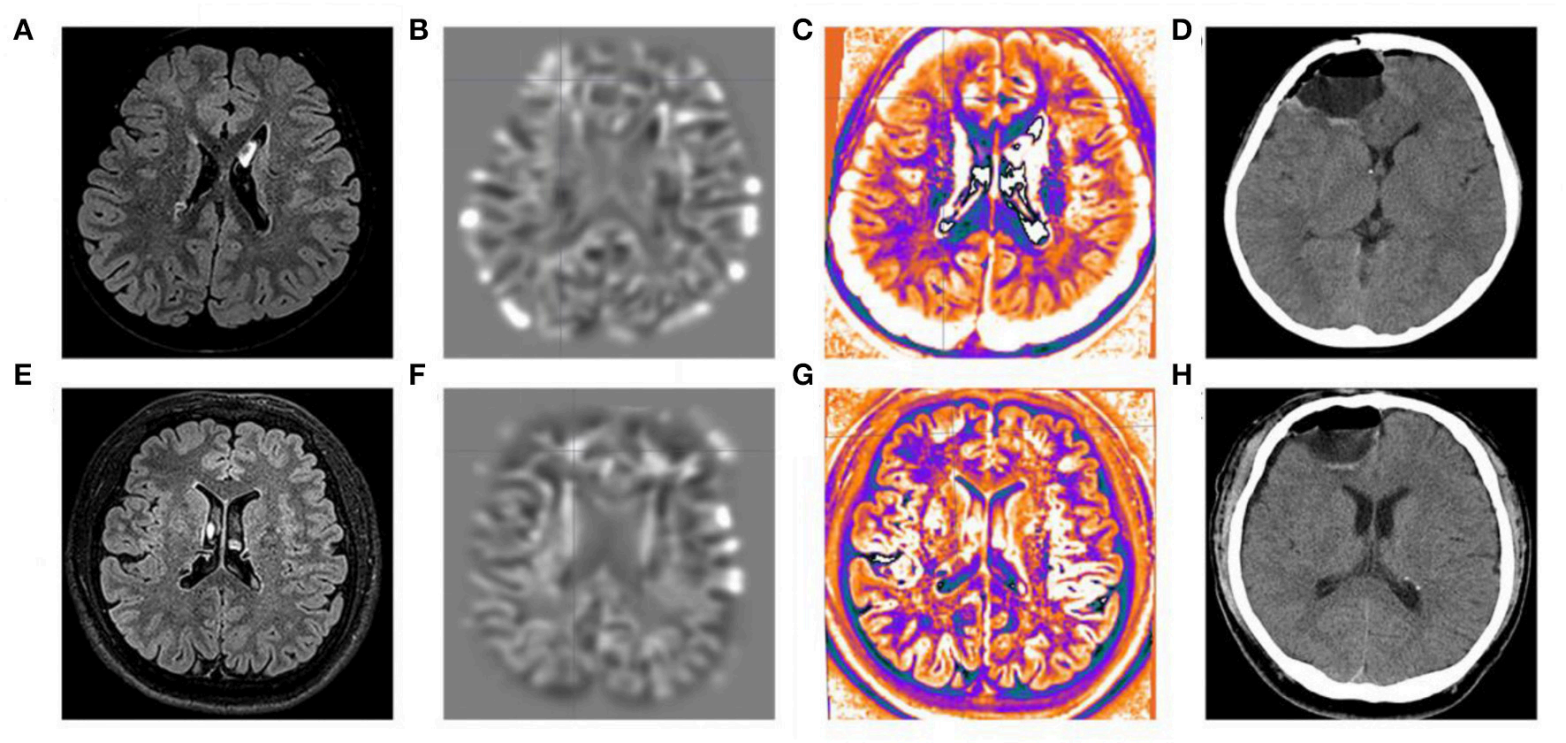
MRI morphological analysis and postoperative CT of case 2 and case 3. The (A) FLAIR, (B) junction imaging, (C) normalized FLAIR signal intensity (NFSI), and (D) postoperative CT of case 2. The (E) FLAIR, (F) junction imaging, (G) NFSI, and (H) postoperative CT of case 3.
